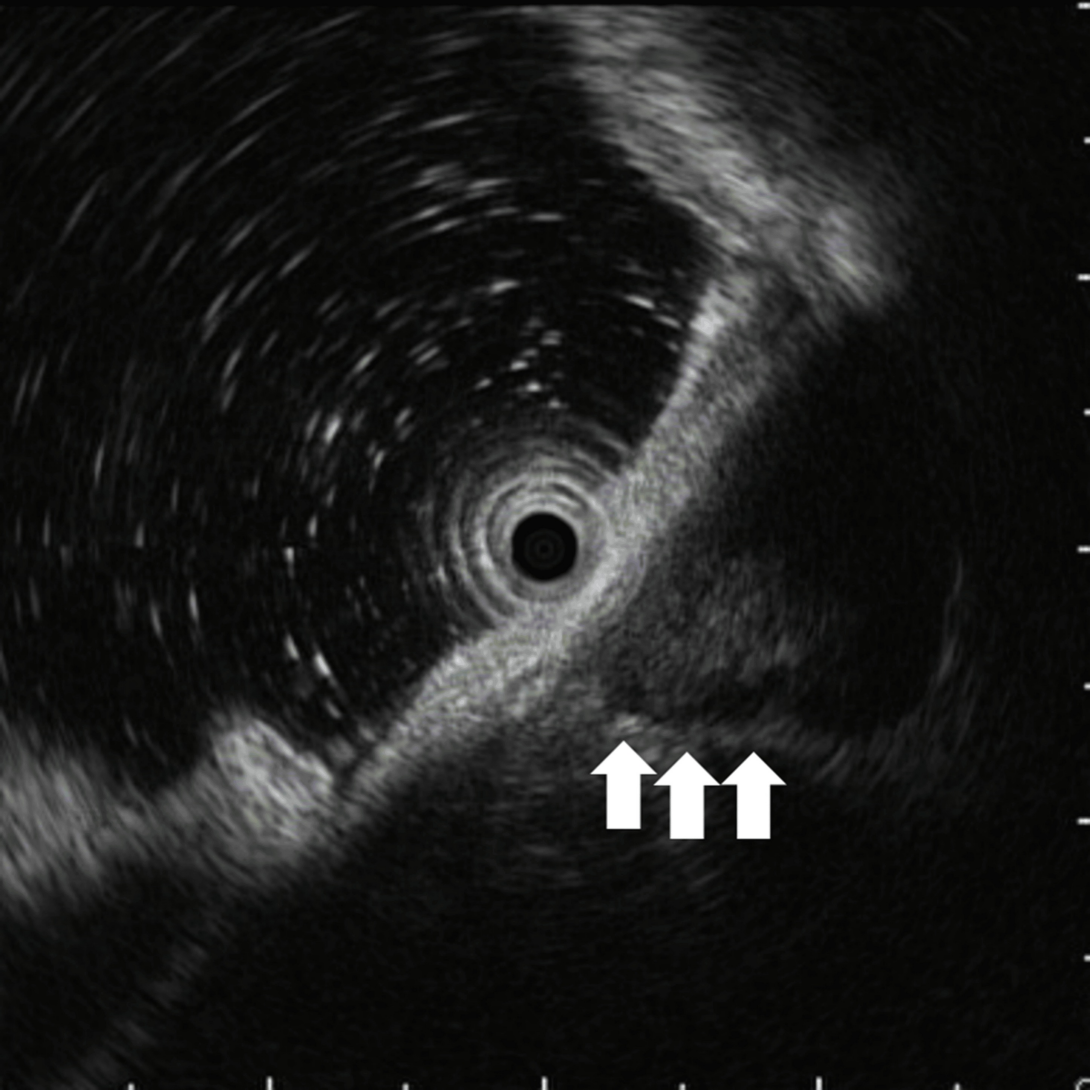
Endoscopic ultrasonography of case 1. Endoscopic ultrasonography showing a heterogeneous internal echo pattern and an irregular tumor margin (arrows), consistent with a gastrointestinal stromal tumor. The tumor originated from the fourth layer of the gastric wall.
